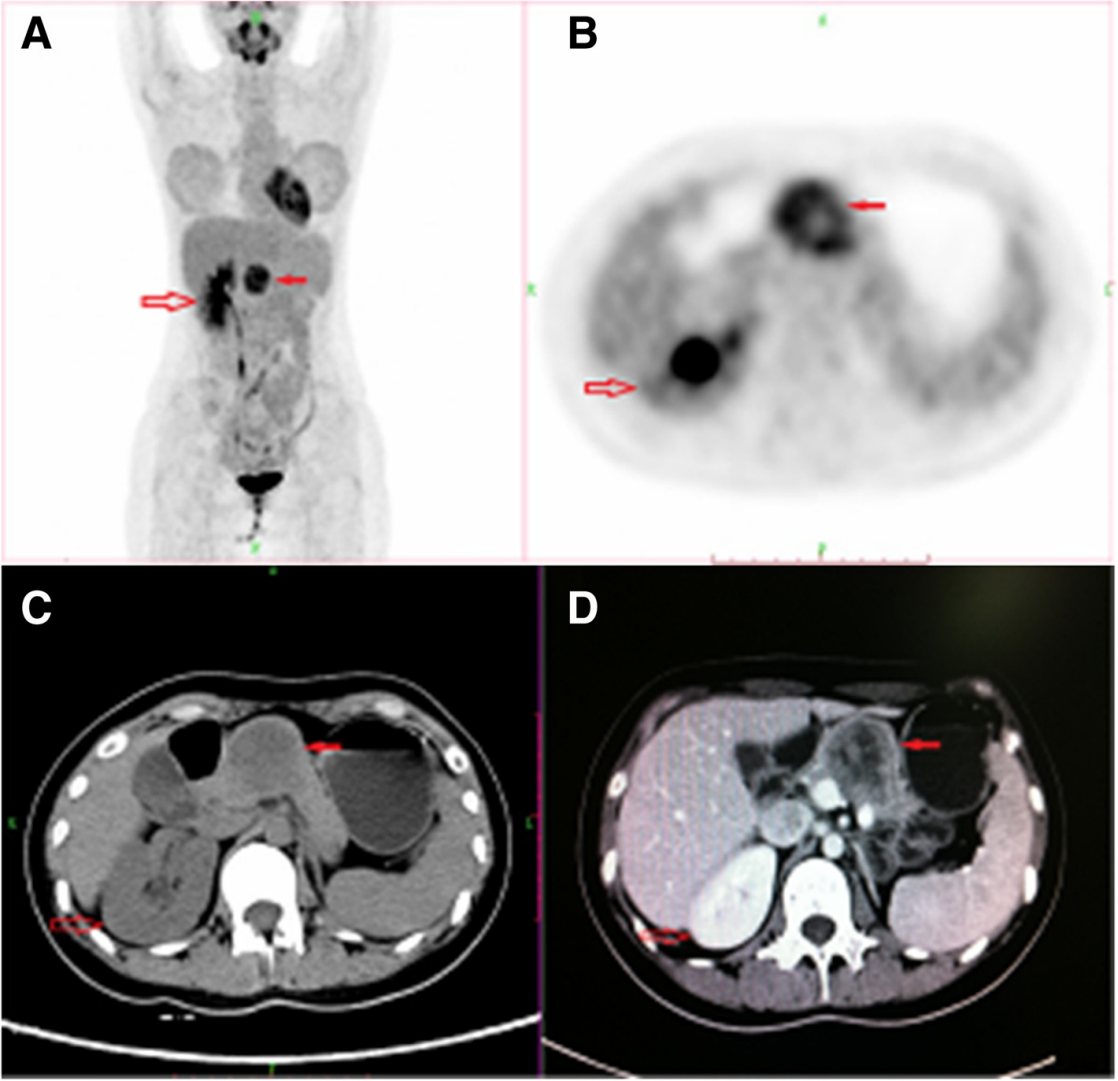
The tumor was located in the body of the pancreas with intense FDG uptake (A-B, solid red arrow). The tumor exhibited hypoattenuation on pre-contrast CT (C), and was enhanced inhomogeneously after contrast administration (D). The FDG accumulation was mainly located in the peripheral part of the tumor (B), corresponding to the solid part of the tumor with enhancement (D). Only the right kidney was found (A-D, red arrow).
